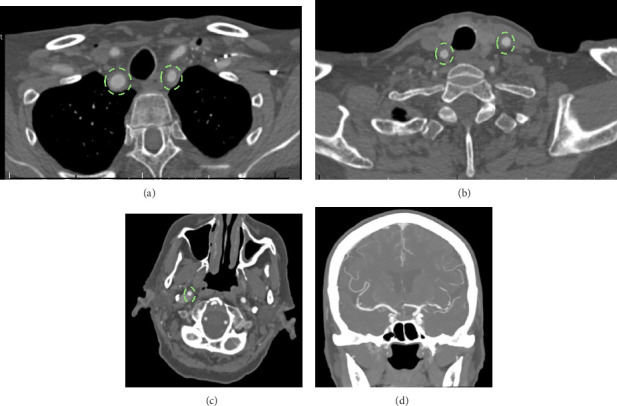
(a, b) CTA head and neck demonstrating diffuse mural thickening of bilateral subclavian and carotid arteries (dotted outline). (c) CTA neck demonstrating mural thickening of the right distal internal carotid artery. (d) CTA head demonstrating bilateral MCA stenosis.
